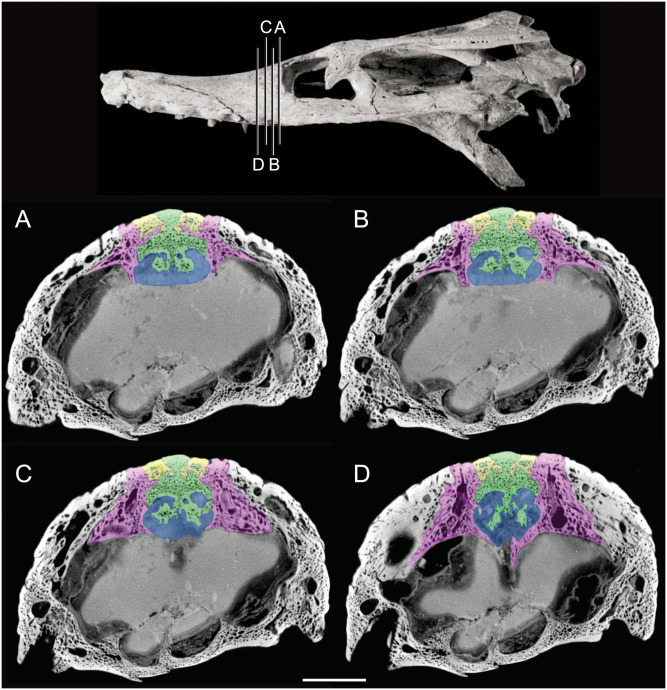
Detail of bony olfactory lamina. Skull of AMNH FARB 33354 at top showing position of coronal CT slices exhibited in A, B, C, and D. Area of olfactory bulb highlighted in blue, frontal in green, nasals in yellow, prefrontals in purple. Scale bar equals 10 mm.
